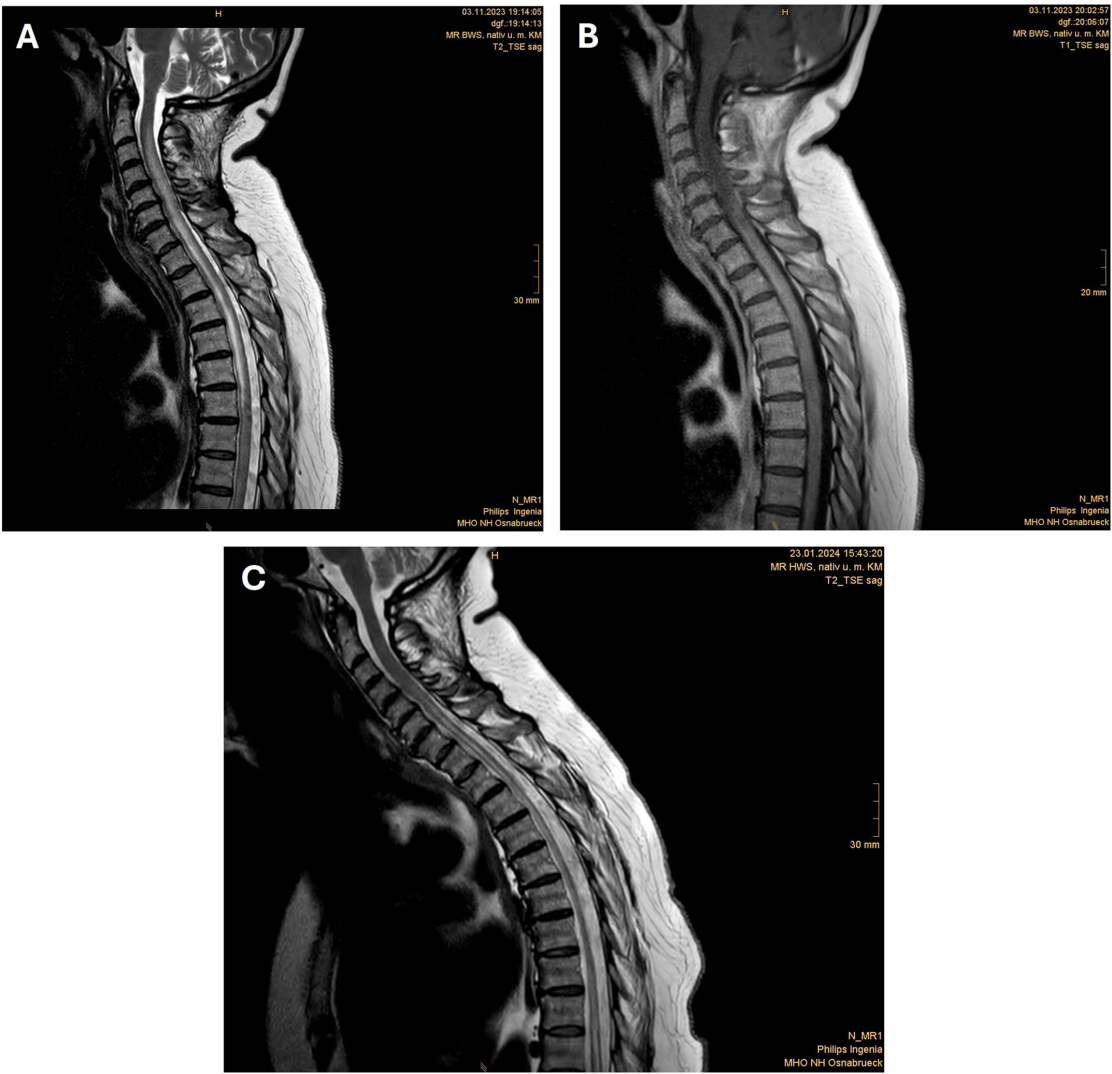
Spine MRI shows longitudinally extensive transverse myelitis from C2 to T5 before ravulizumab initiation in T2W (A) and T1W with gadolinium enhancement (B) and regression of the spinal cord edema but persistent longitudinal atrophy after ravulizumab initiation in T2W (C).
